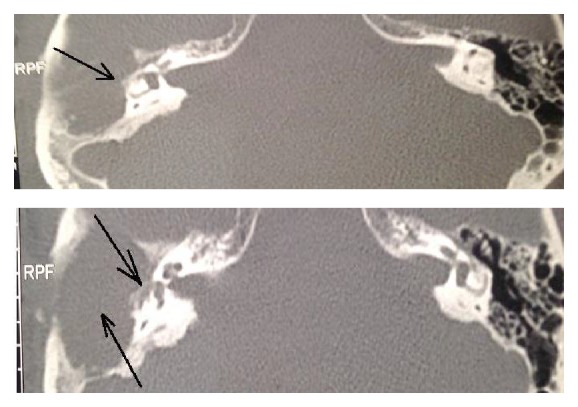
Case 3. Two different slices of the CT scan showing the fistula of the right horizontal semicircular canal and the middle ear filled with cholesteatoma and inflammatory tissue.
